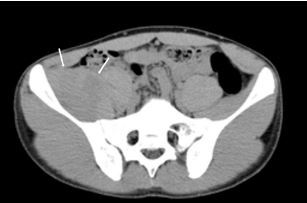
Contrast-enhanced computed tomography showing the enlarged right iliacus muscle (arrows).
